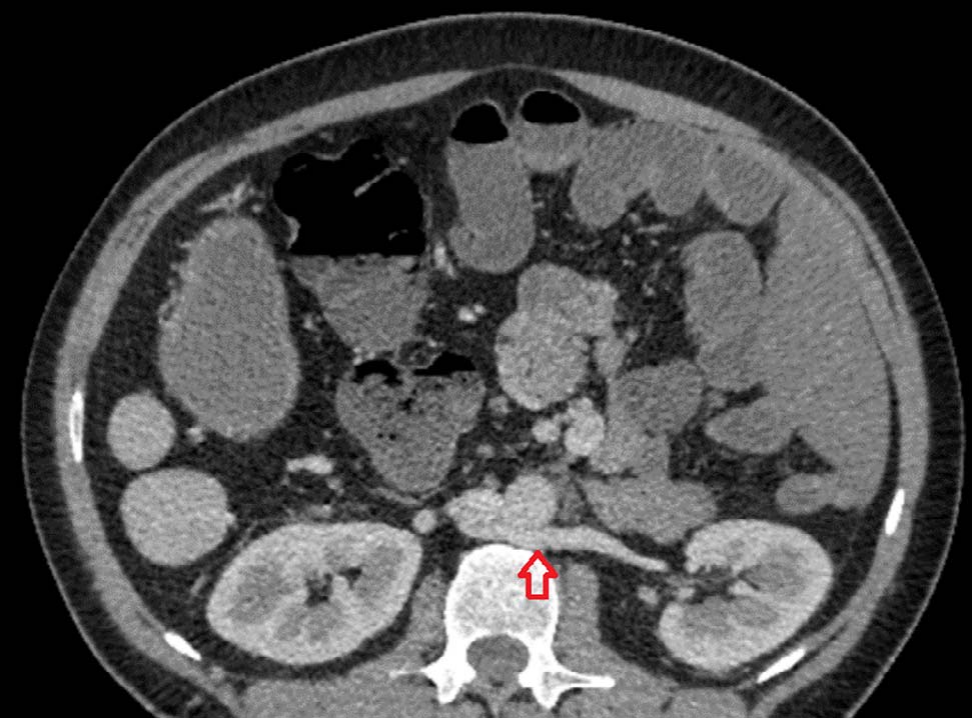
Contrast-enhanced axial fat-saturated computed tomography image shows the retroaortic course of the left renal vein (red arrow).
